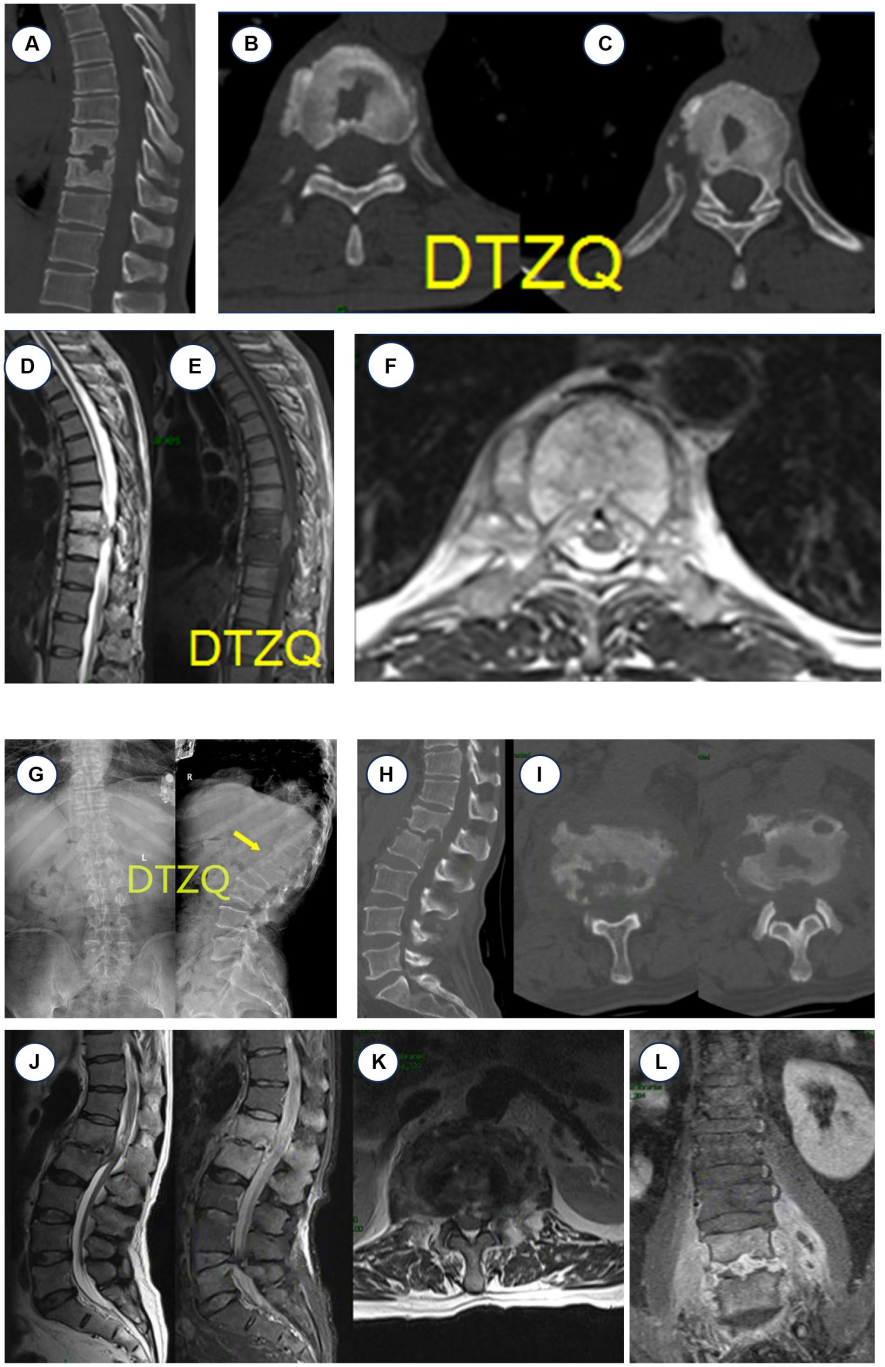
Case 1 CT and MRI manifestations of thoracic brucellosis spondylitis (T9–T10). (A–C) CT: T9, T10 vertebral bone destruction, intervertebral space narrowing. (D–F) MRI: T9–T10 vertebral body signal abnormality, vertebral body destruction with abscess, thoracic spinal cord compression. Case 2 X-ray, CT, and MRI findings of brucellosis spondylitis. (G) X-ray L1–L2 intervertebral space significantly narrowed. (H,I) CT showed worm-like bone destruction at L1–L2. (J–L) MRI showed inflammatory changes in the disk and vertebral body at L1–L2, abnormal high signal intensity, narrowing of the disk space, abscess formation, inflammatory tissue protruding into the spinal canal, and spinal cord compression.
